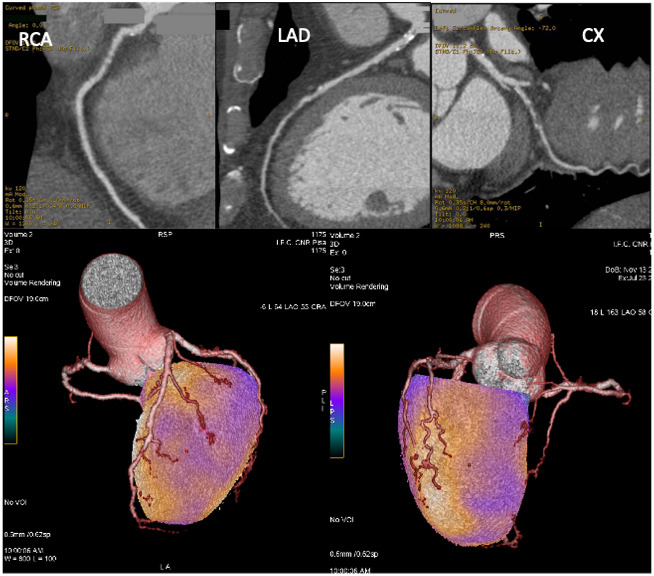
Coronary CT angiography and hybrid myocardial perfusion
